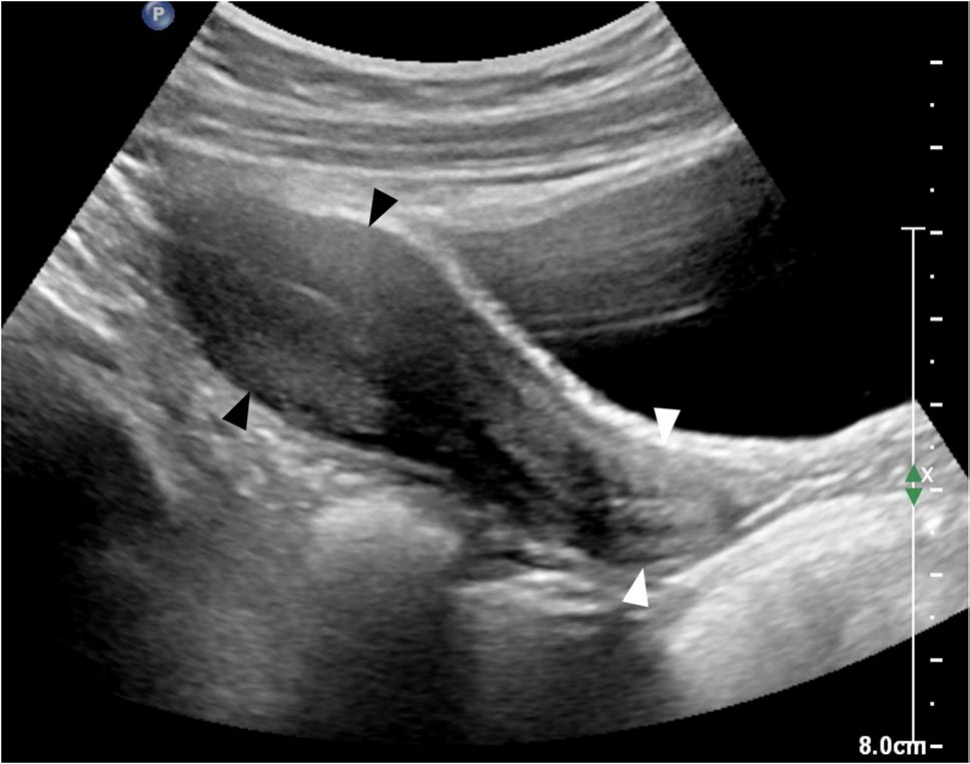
Sagittal gray-scale pelvic ultrasound image in a 13-year-old girl shows a mature pear-shaped uterus: the fundus (*black arrowheads*) is now wider than the cervix (*white arrowheads*)
